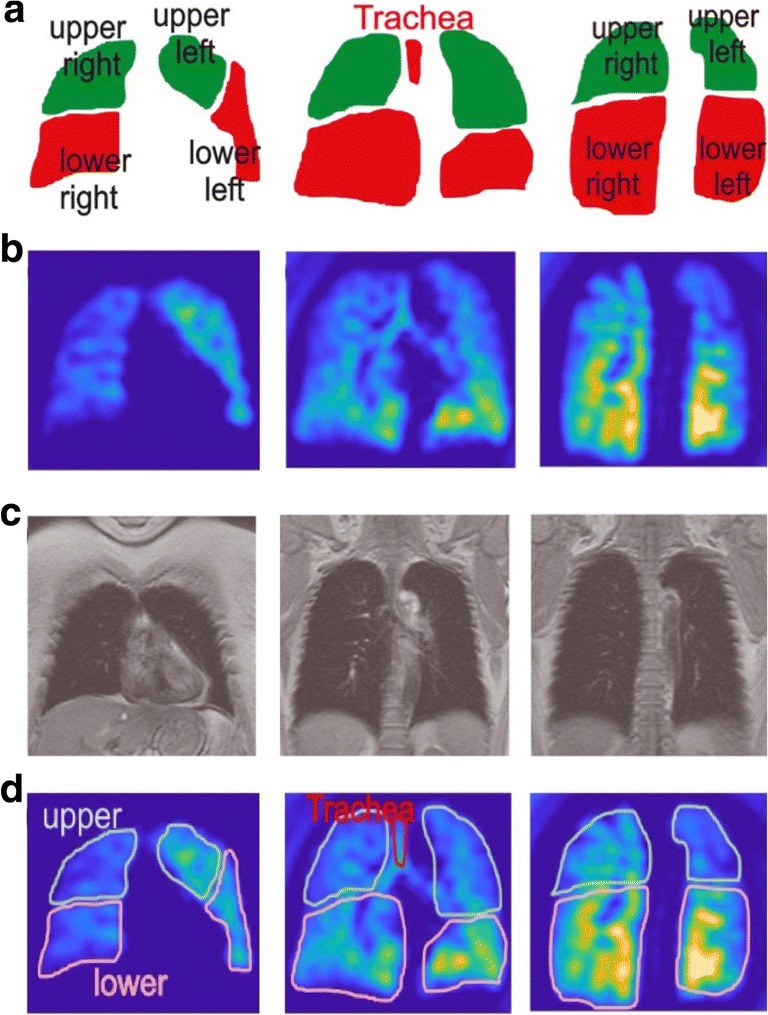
Representative regions of interest (ROIs), as manually specified on coronal slices; outlining the trachea, and upper and lower areas of each lung. a Region of interest mask contours. b Two interleaved high-temporal resolution DXeV-MRI images. c Corresponding ^1^H-MRI images. d User-defined tracheal and lung ROIs on DXeV-MRI images
